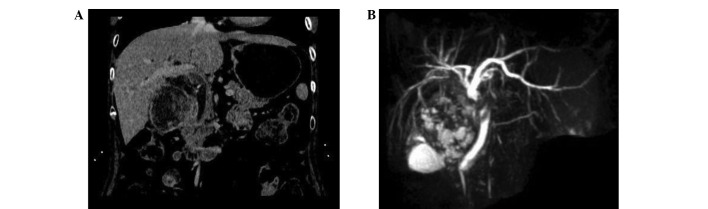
Pre-operative examination. Three-dimensional reconstruction by (A) CT scan and (B) magnetic resonance cholangiopancreatography revealed enlargement of the gallbladder with a large mass and tumor thrombi presenting as soft tissue mass in the common bile duct, with biliary dilatation above the obstruction. CT, computed tomography.
